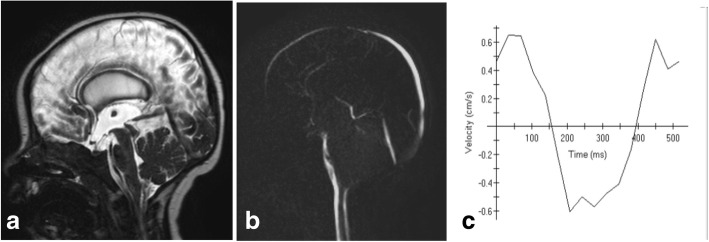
A 3-month-old boy with hydrocephalus. a Sagittal 3D-DRIVE demonstrates aqueduct stenosis. b Phase-contrast MRI shows absence of detectable flow at the region of the aqueduct with markedly diminished velocity (c)
